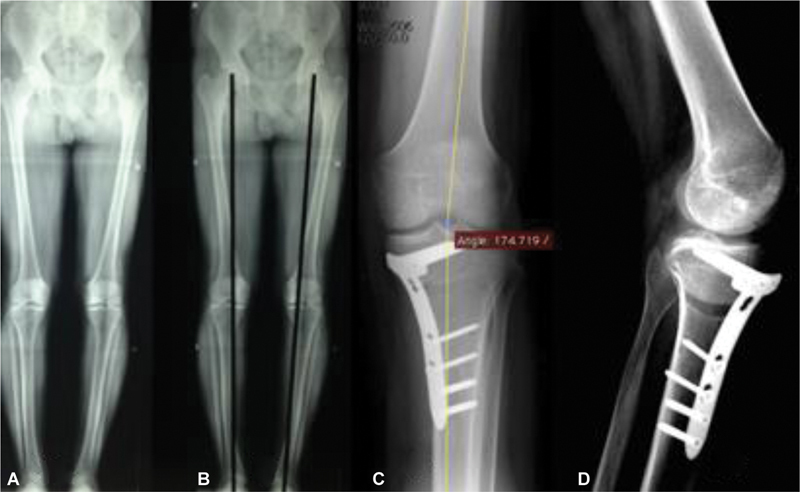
Panoramic radiographs of the lower limbs showing a slight mechanical axis in varus (
